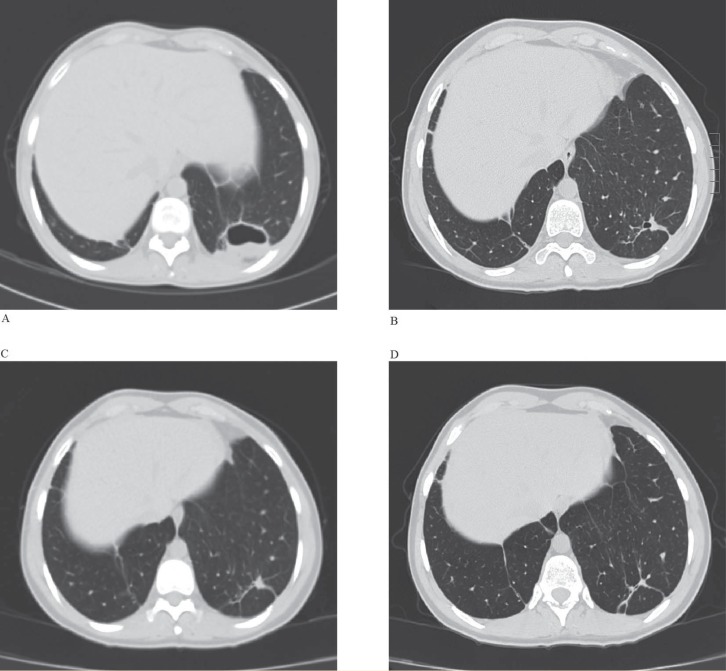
CT scans of the lung abscess regression over the treatment period. A) Lung abscess of the left lung after two months of beginning of the treatment (4/2015). B) Beginning of abscess resolution (7/2015). C) Complete resolution of the abscess (10/2015). D) Intrathoracic status a year after the treatment (3/2016
